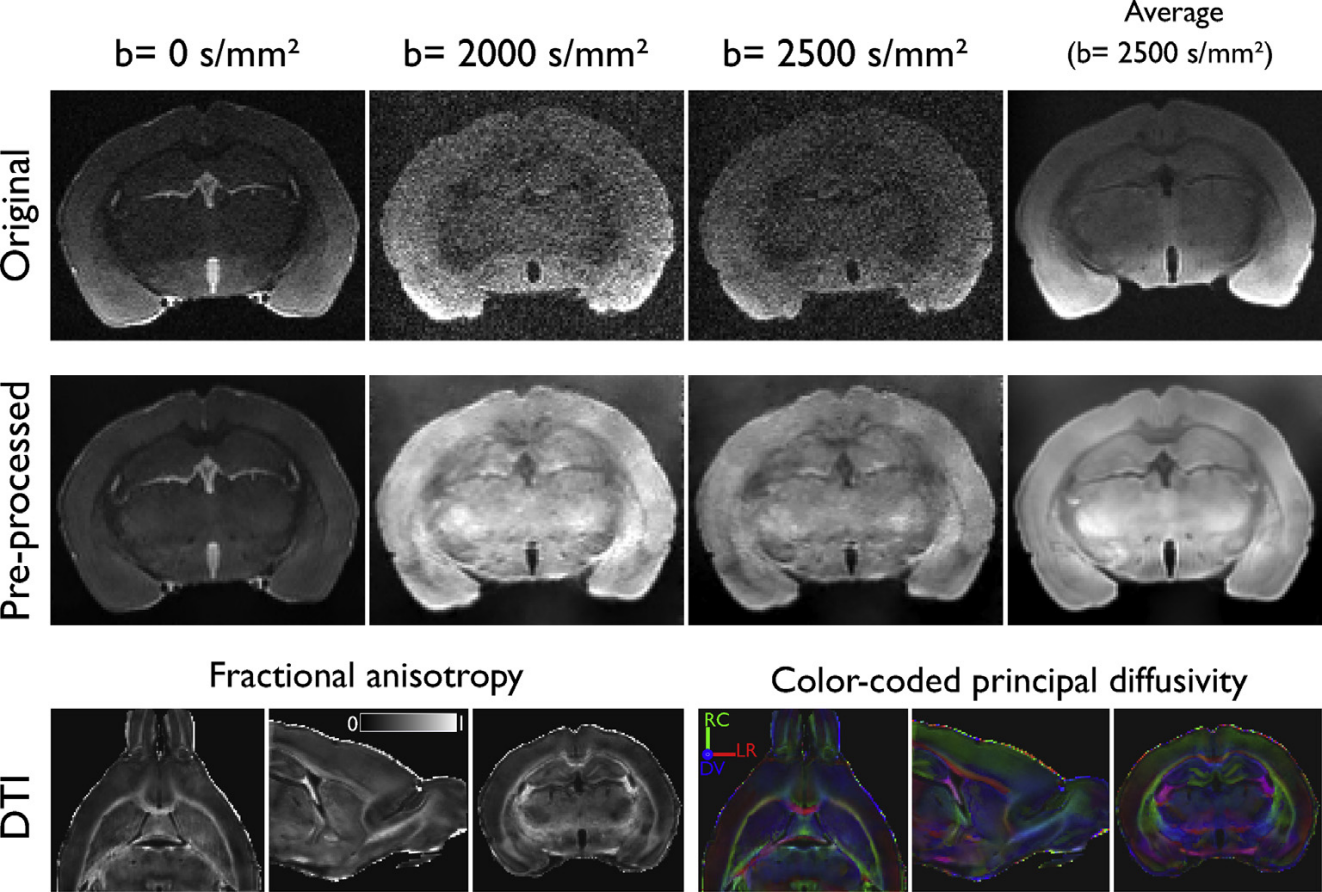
Example *ex vivo* DWI images, before and after pre-processing, and derived diffusion tensor imaging (DTI) maps. RC: rostro-caudal; LR: left-right; DV: dorso-ventral.
